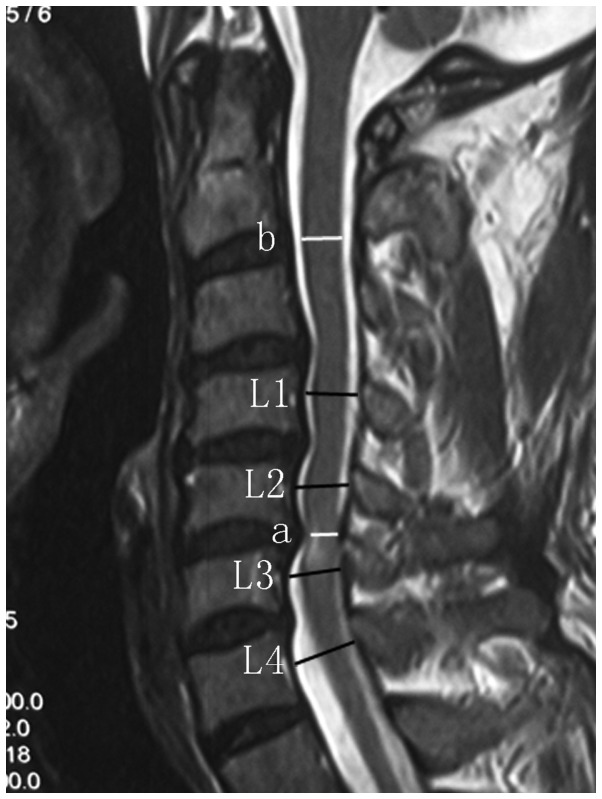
Measurement of diameters of the cerebrospinal fluid (CSF) column and spinal cord compression on T2 sagittal MRI. L1–L4 were diameters of the CSF at the mid-vertebra level from C4 to C7. Diameter of the CSF column (mm) = (L1+L2+L3+L4)/4. a and b are the spinal cord diameters of narrowest part and the C2/3 intervertebral level. Spinal cord compression (%) = a/b.
